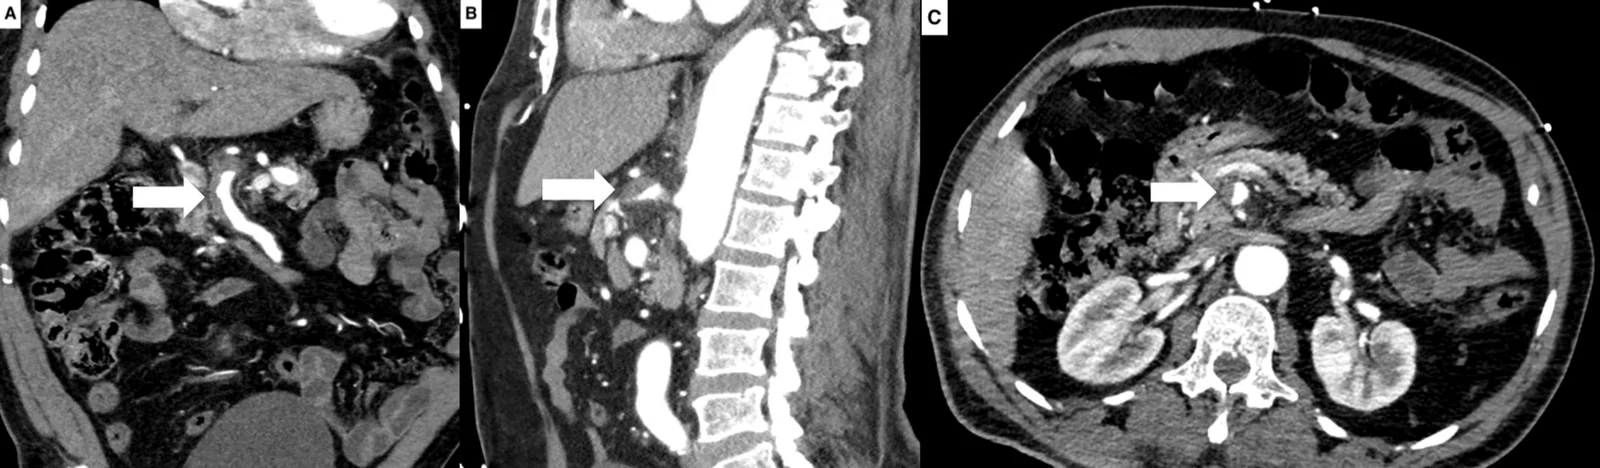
Initial CTA of the abdomen and pelvis on the day of admission demonstrating a superior mesenteric artery dissection measuring approximately 4 cm in length. (A) Coronal, (B) sagittal, and (C) axial views showing the dissection with associated mild-to-moderate luminal narrowing CTA: computed tomography angiography
